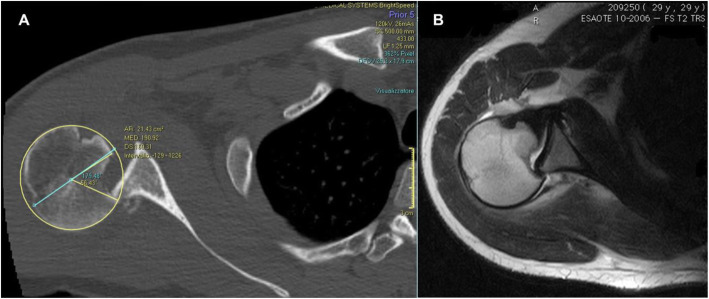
The defect angle (DA) and the Cartilage Angle (CA) are calculated on a CT slice taken at or immediately below the coracoid tip. The percentage of the CA (179° in this case) that the DA (56° in this case) represents is the estimated size of the defect (31% in this case)
